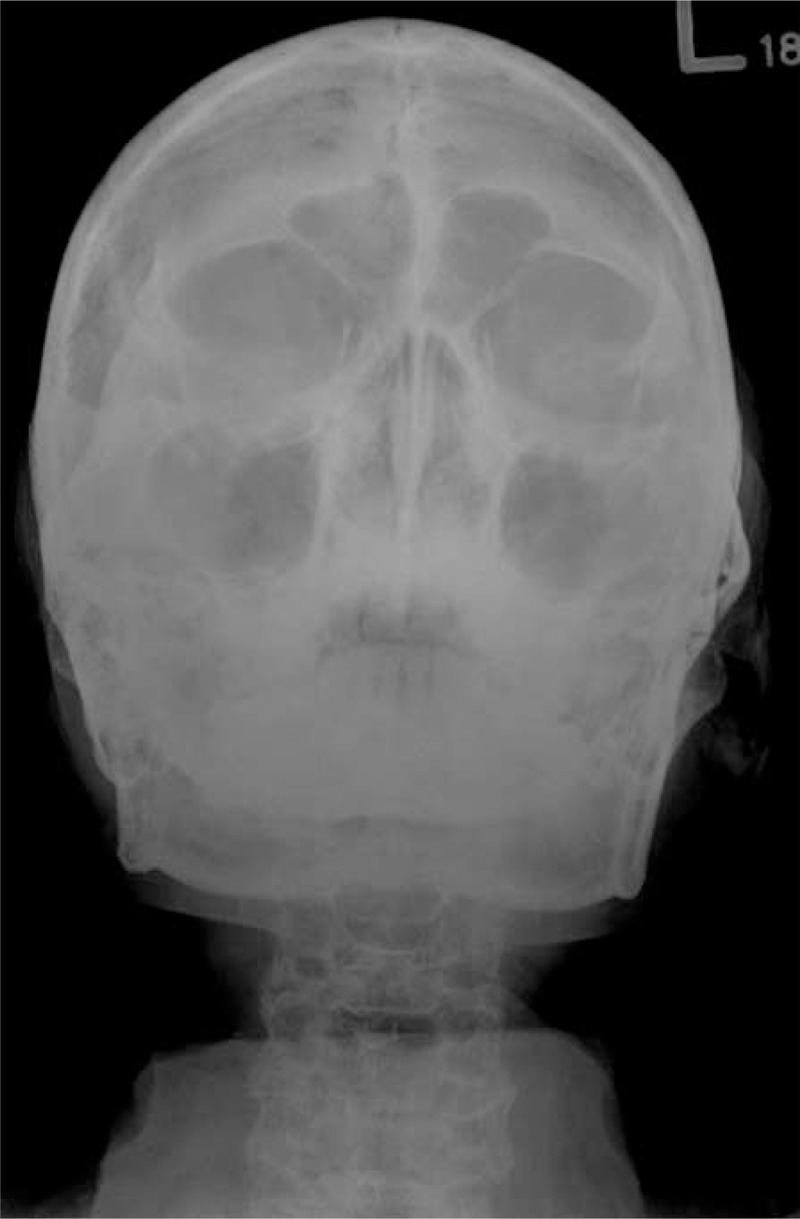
Water's view of skull X-ray was arranged for possible sinusitis, which showed clear paranasal sinuses, except multiple punch-out lesions on skull.
